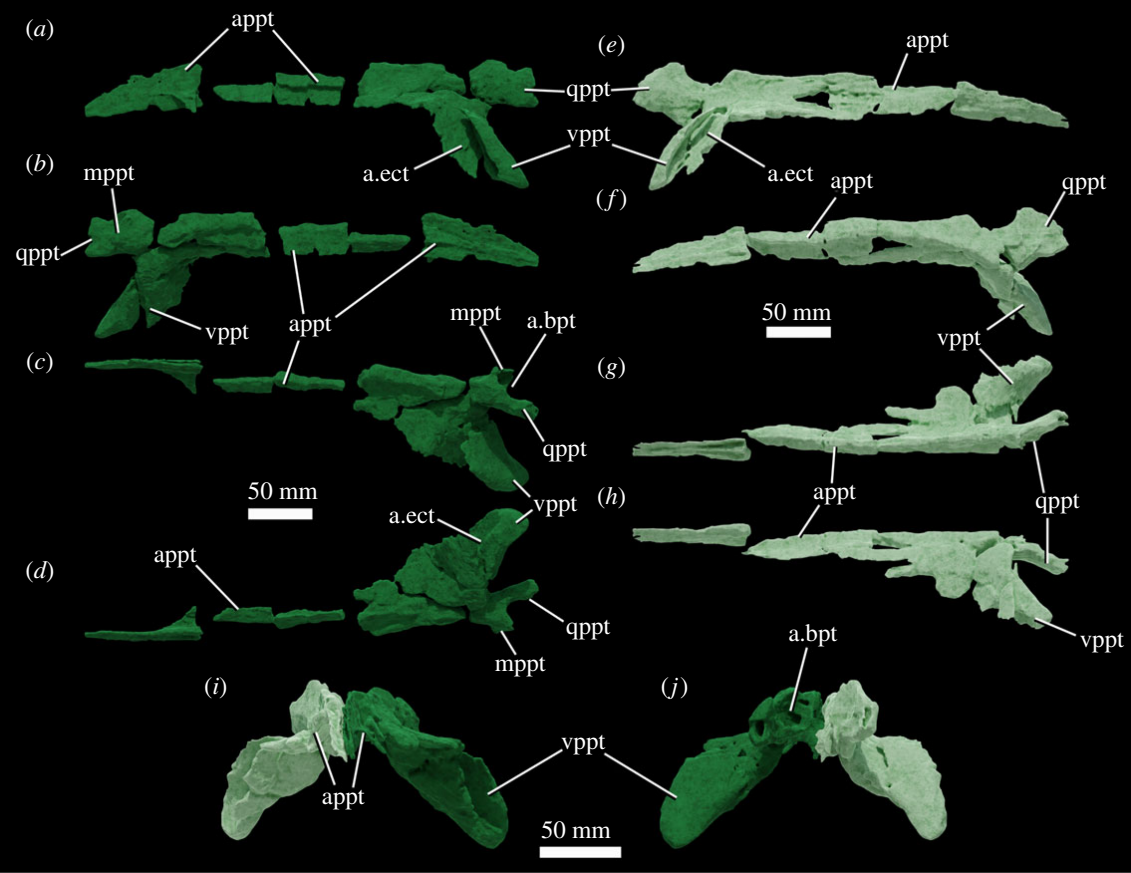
Pterygoids of NHMUK R36620, holotype of *Mambawakale ruhuhu*, reconstructed from CT data. Left pterygoid in lateral (*a*), medial (*b*), dorsal (*c*) and ventral (*d*) views. Right pterygoid in lateral (*e*), medial (*f*), dorsal (*g*) and ventral (*h*) views. Paired pterygoids in anterior (*i*) and posterior (*j*) views. Abbreviations: a.bpt, articular surface for the basipterygoid process of the basisphenoid; a.ect, articular surface for ectopterygoid; appt, anterior process of the pterygoid; mppt, medial process of the pterygoid; qppt, quadrate process of the pterygoid; vppt, ventral process of the pterygoid.
